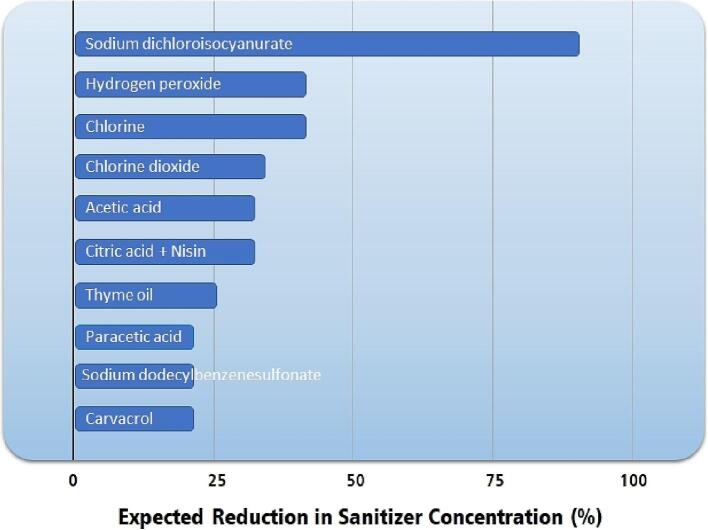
Estimated reduction in sanitizer concentration attained by ultrasound application during the initial washing and sanitizing stage of fruits and vegetables. Data were obtained in the literature [61], [63], [64], [65], [68], [69], [71], [55].
